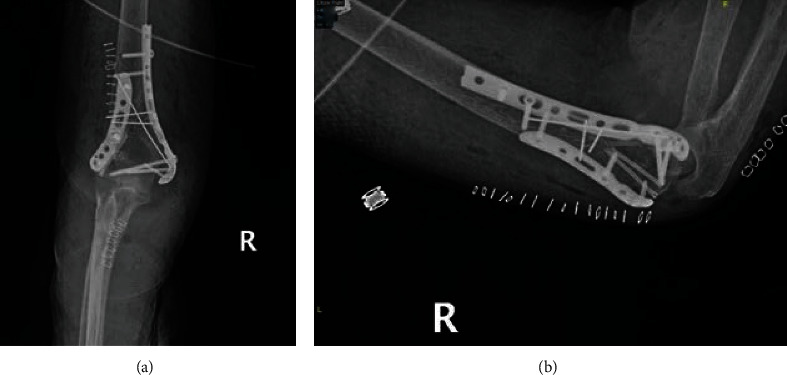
Postoperative plain radiographs of the right elbow showing on the (a) AP and (b) lateral views reduction of the medial and lateral epicondyles using double plating with ten screws.
